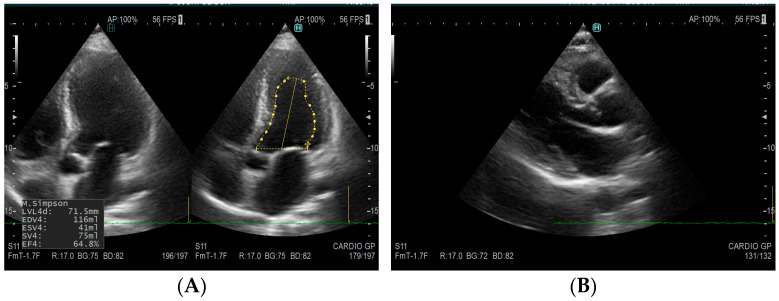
Transthoracic echocardiographic images of normal valves. (A) Apical five-chamber echocardiographic view demonstrating normal mitral and aortic valve anatomy. (B) Parasternal long-axis echocardiographic view showing normal mitral and aortic valves. Source: Images obtained at Spitalul de Urgență Deva, Romania. Used with institutional permission.
